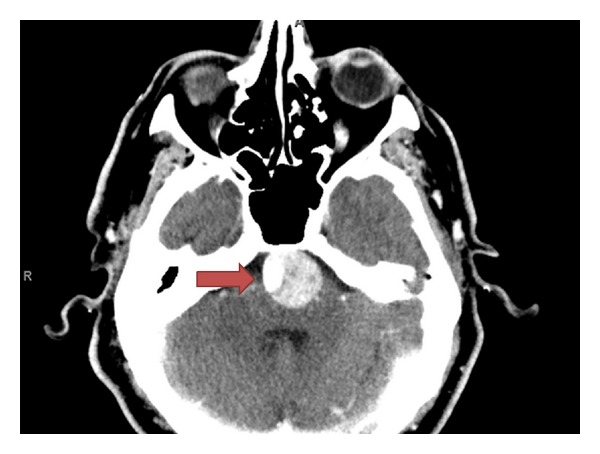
Head CTA: There is a very large aneurysm of the basilar artery measuring 25 mm in diameter, which shows a large amount of thrombus within it. The right lateral aspect of the thrombus remains canalized, extending into the basilar tip. There is associated mass effect on the medulla and pons. There is no evidence of aneurysm rupture.
